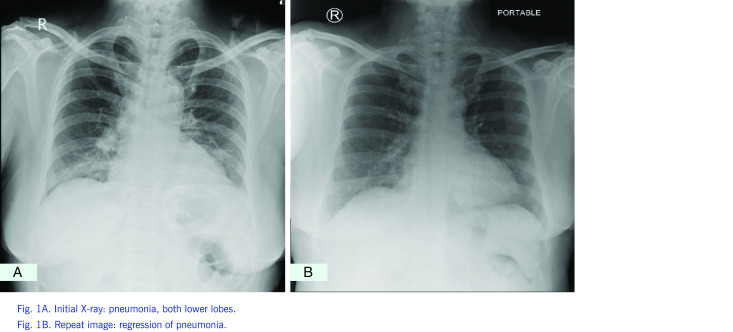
Chest radiographic image
